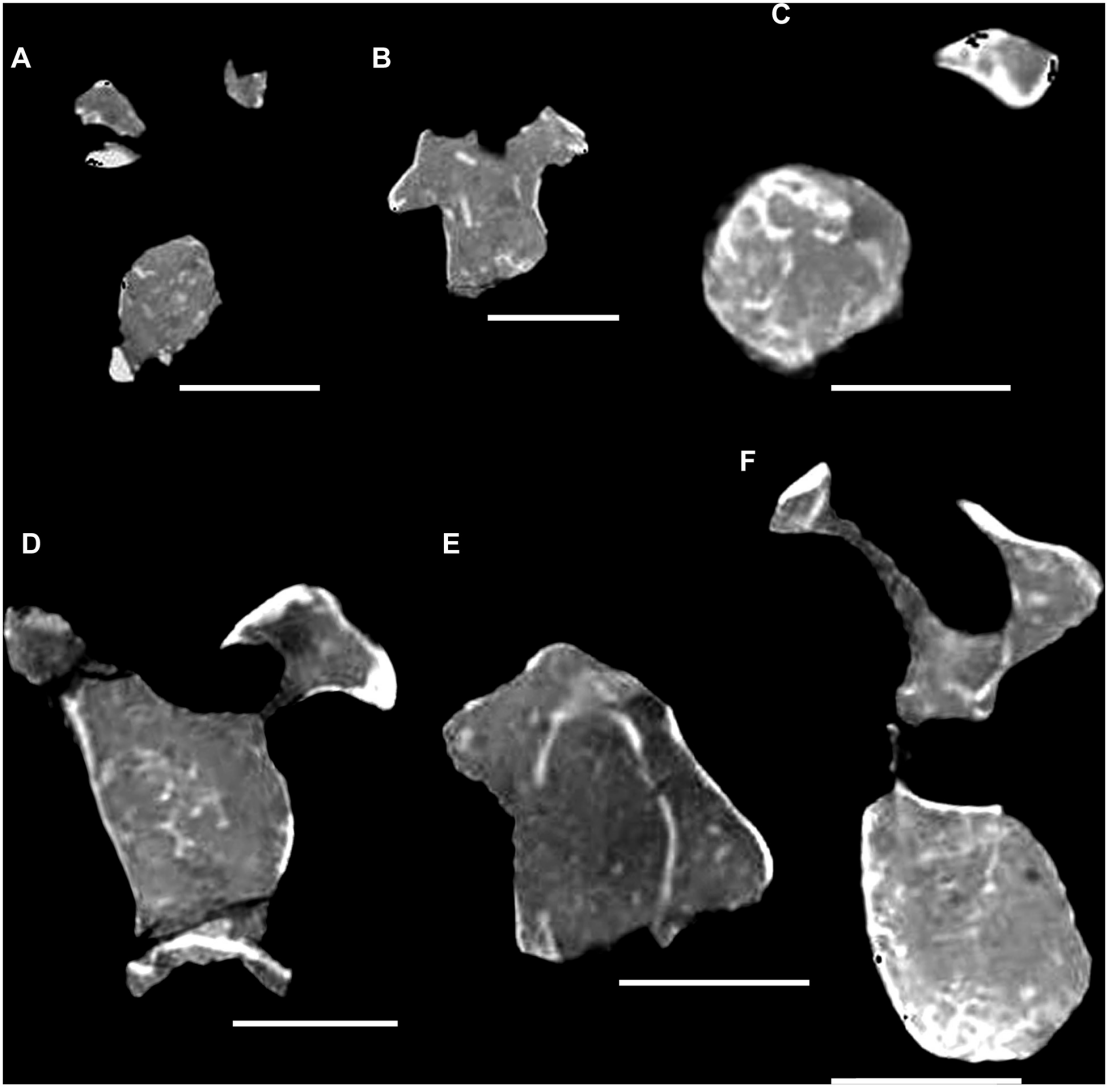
Computed tomographic transverse sections through cervical elements of *Sarmientosaurus musacchioi* gen. et sp. nov. (MDT-PV 2), showing extensive pneumaticity. (A) Axis and associated rib. (B) Cervical vertebra 3. (C, D, E) Cervical vertebra 6. (F) Cervical vertebra 7. Scale bars = 5 cm.
